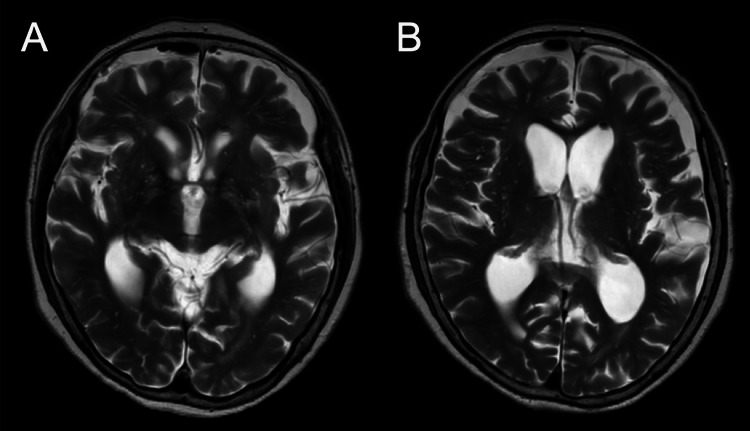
T2-weighted images obtained seven days after the surgical intervention. The ependyma of the right lateral ventricle was broadly covered with membranous structures. Sections through the third ventricle (A) and the lateral ventricles (B) Ventricular enlargement was alleviated
